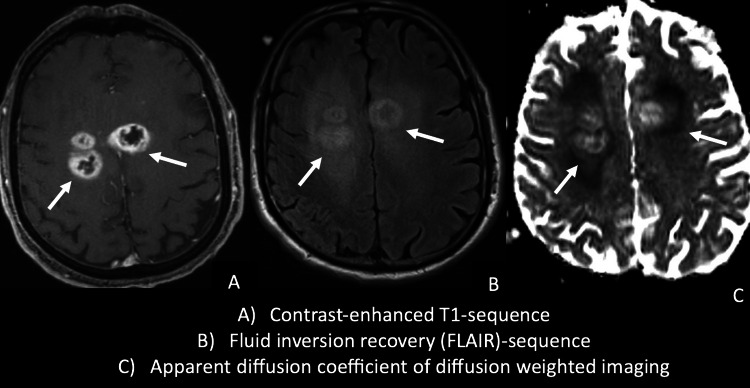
Example of a patient with mGB. A Contrast-enhanced T1 sequence showing three separate tumor lesions. B Fluid inversion recovery (FLAIR) sequence demonstrating the tumor infiltration zone within the perilesional region. C Diffusion weighted imaging (DWI) with the apparent diffusion coefficient (ADC) showing higher ADC values within the tumor lesions but lower ADC values within the perilesional regions
